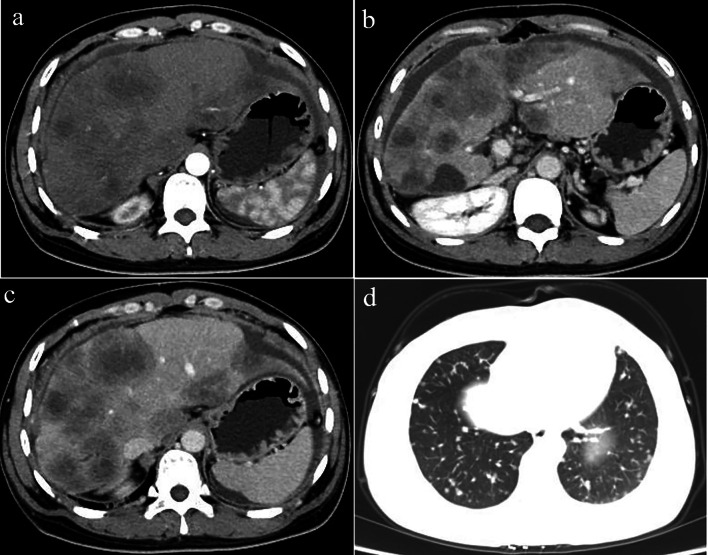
A 34-year-old female with diffuse nodular type HEH. Axial and multiple phase contrast-enhanced CT images (a–c) show multiple, different-sized, coalescing, homogeneous, hypoattenuating nodules diffusing throughout the liver parenchyma. Masses show ring-like enhancement and the “target” sign. At the same time, there was arc-shaped effusion around the liver and peritoneal thickening. Peripheral distribution of masses and capsular retraction are also observed. The axial lung window image (d) shows lung involvement consisting of multiple round-like nodules
